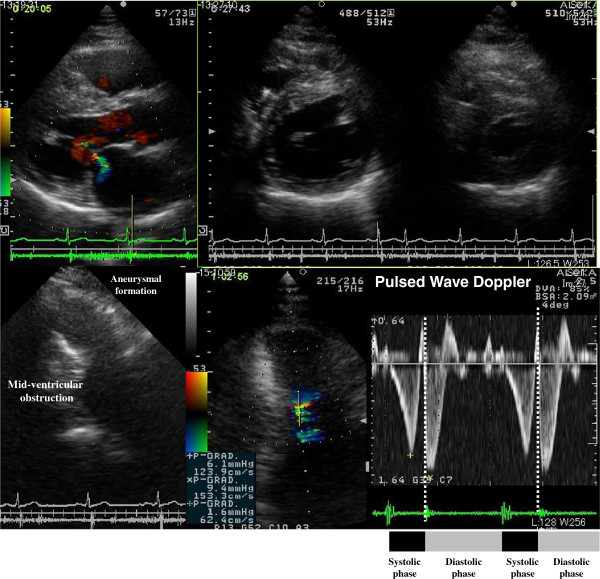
Echocardiographic images demonstrate asymmetrical septal hypertrophy and mid-ventricular obstruction associated with paradoxical diastolic flow.
